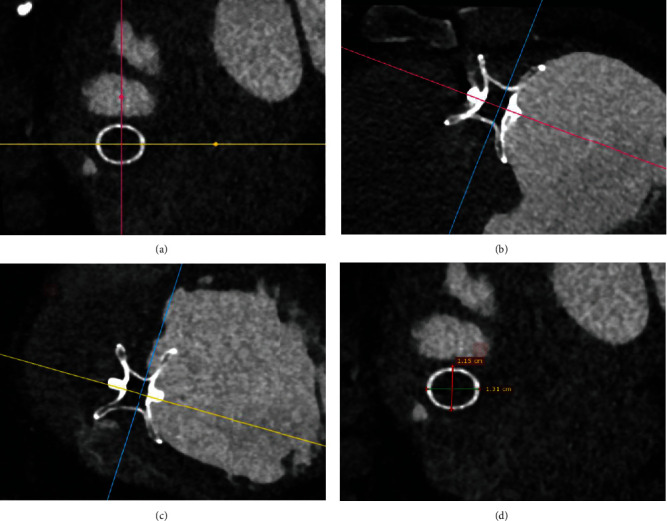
Measurement of POD by 3D-MPR mode of cardiac CT. (a) The finest part of waist in the short-axis section of occluder. (b, c) The long-axis sections of occluder perpendicular to each other. (d) The measurement method of POD, which is the average value of diameter in two vertical directions of occluder in (a).
